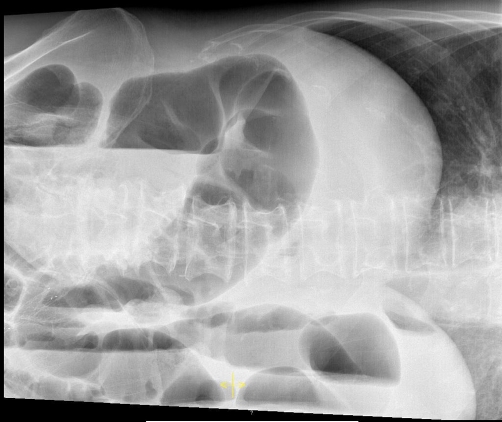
Plain abdominal radiography of patient 2: Distension of the large bowel and gas-fluid-levels as signs for a large bowel obstruction.
